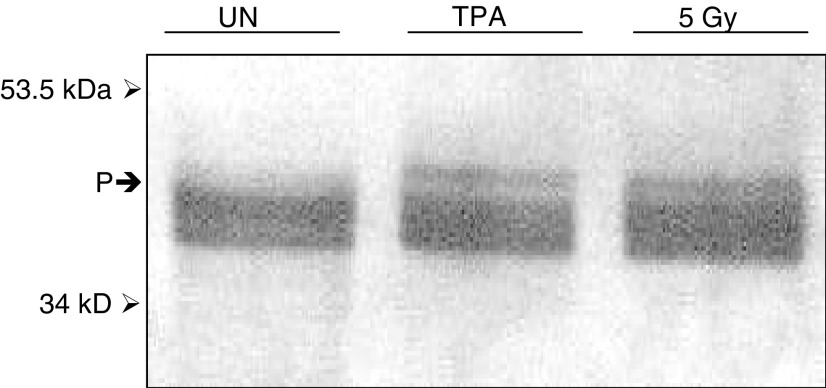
Western blot showing hyperphosphorylation of Cx43 in WB-F344 cells exposed to 5 Gy ultrasoft X-rays (5 Gy) and 20 nM TPA. (P) Demarcation of the band showing phosphorylation in irradiated and TPA-treated cells and the absence of such a band in untreated cells (UN).
